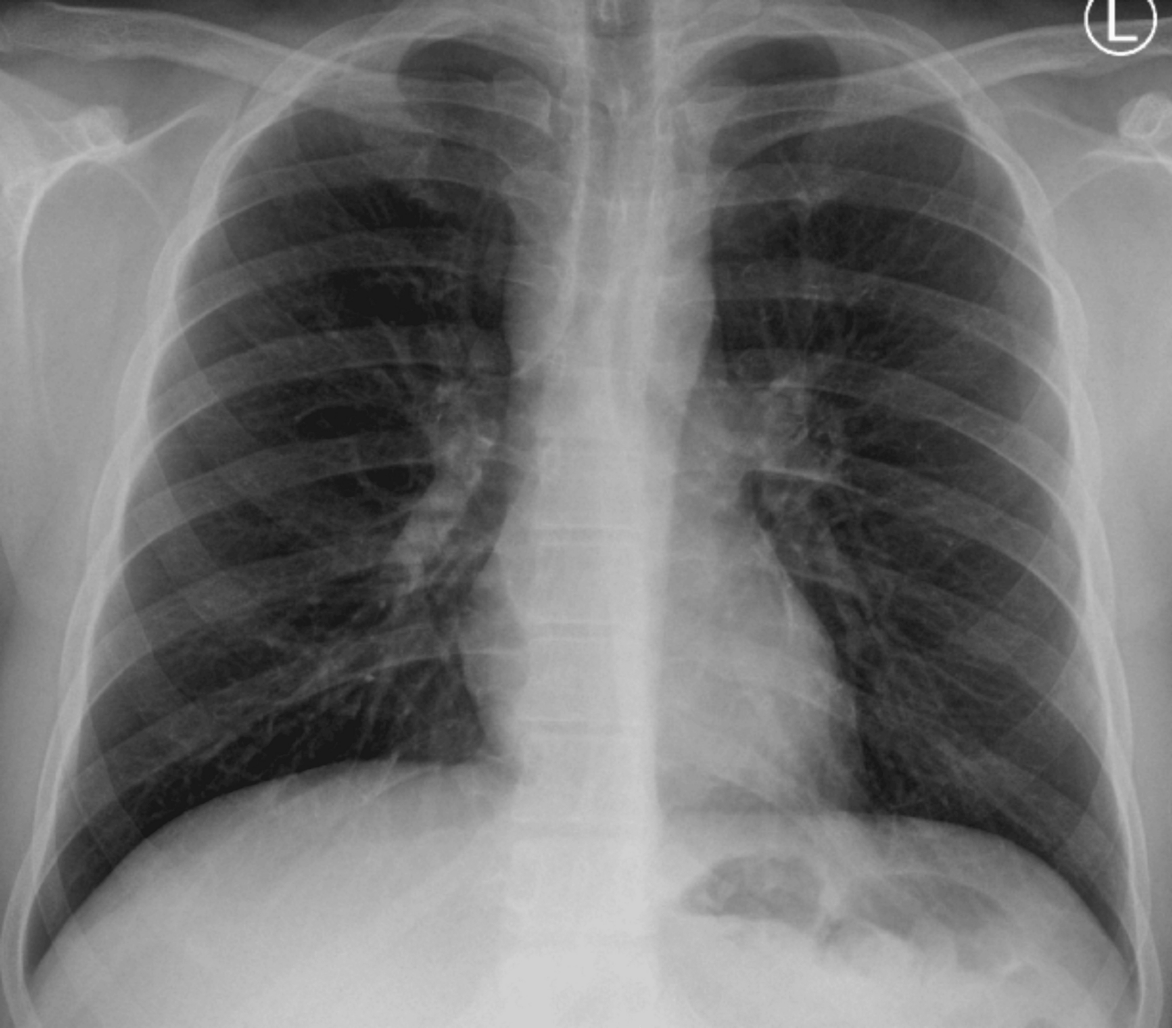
Chest radiograph showing normal appearance of the lungs
